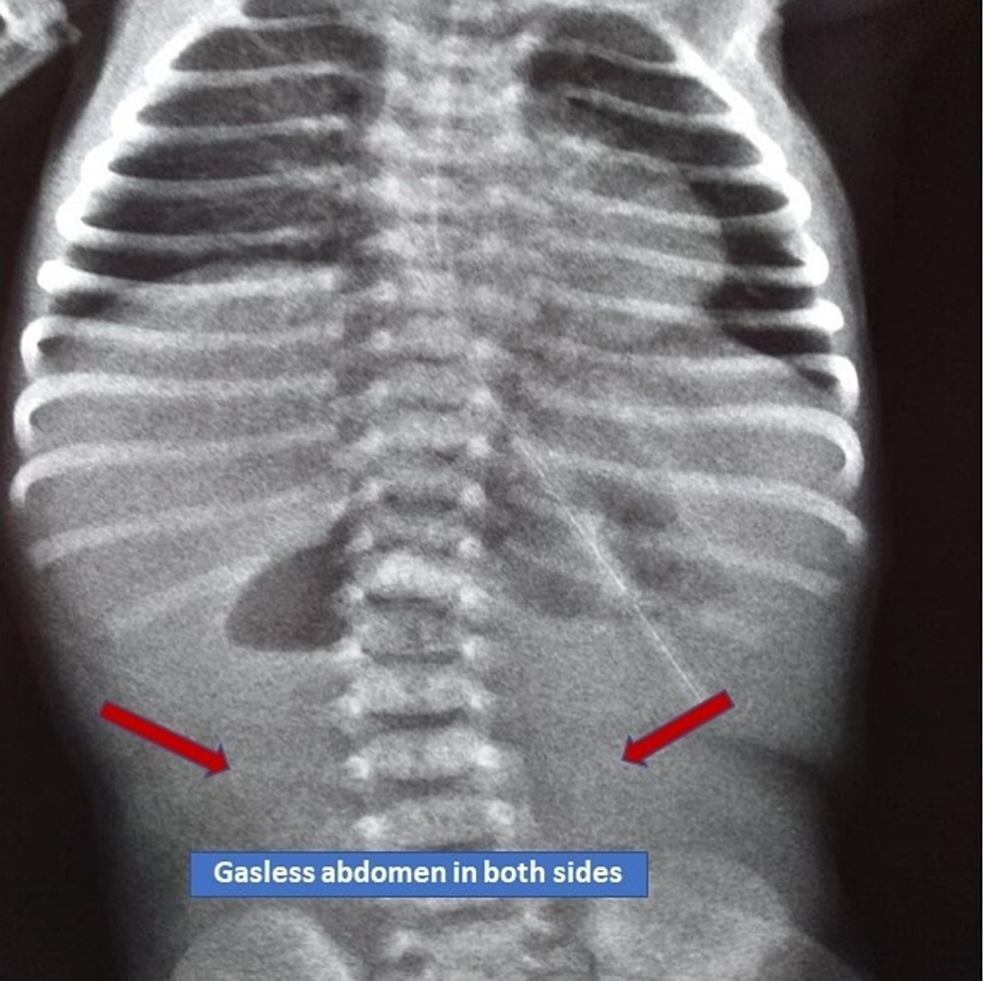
X-ray of abdomen PA view showing paucity of gas abdomen Paucity of gas abdomen is a sign of high small intestinal obstruction.
